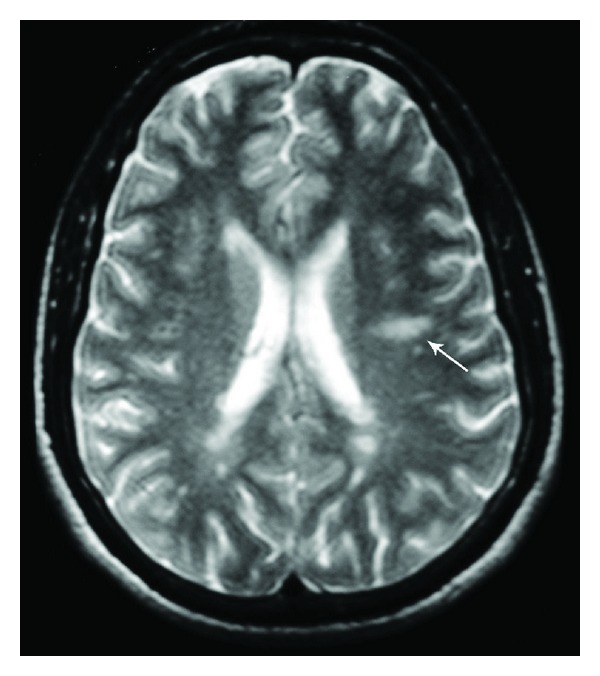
Axial cerebral T2-weighted image showing multiple lesions in periventricular and subcortical white matter. Dawson's finger (arrow) is a characteristic finding in multiple sclerosis.
